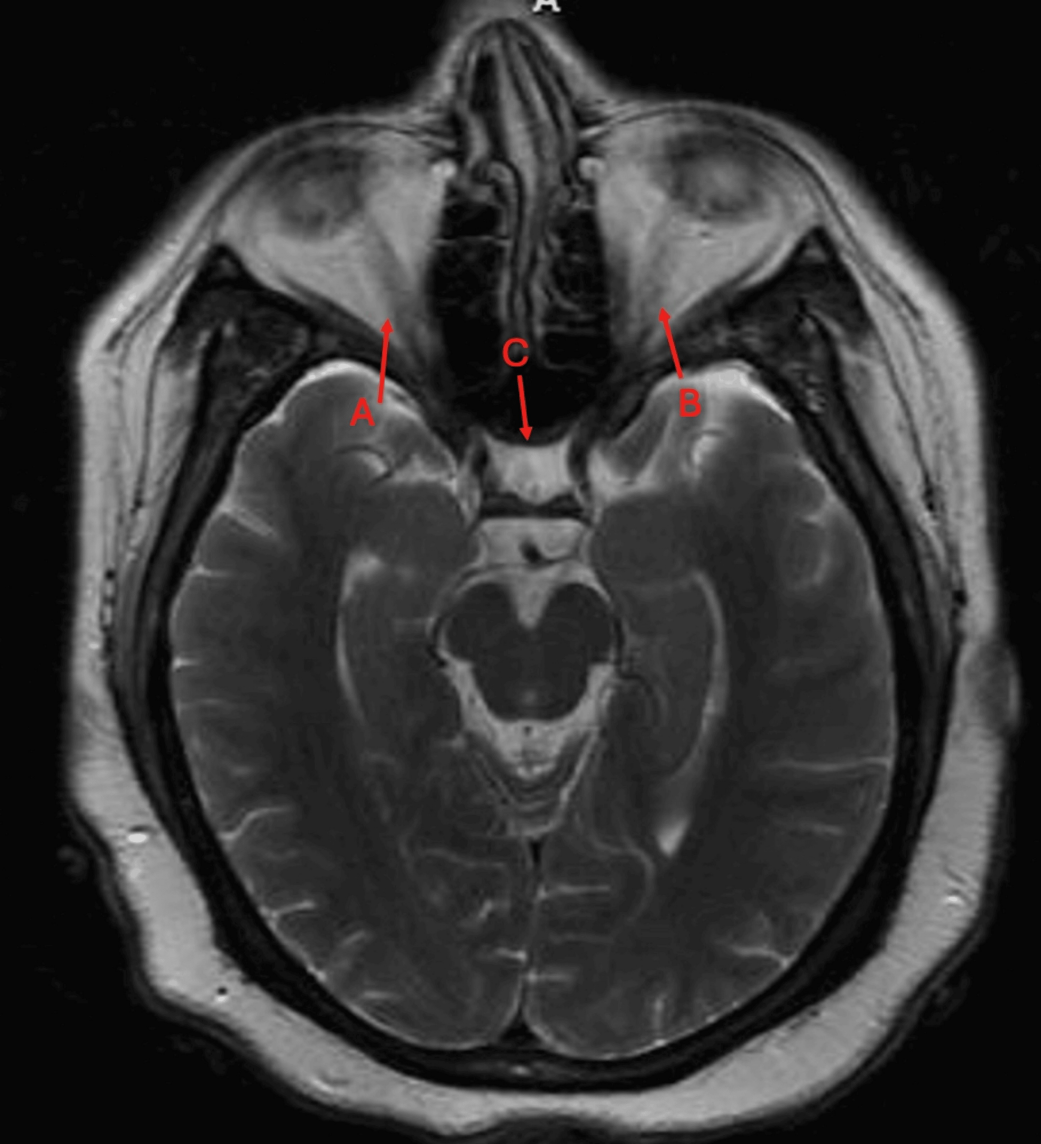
Axial T2-weighted MRI of the brain in a patient with neuromyelitis optica. Arrows A and B indicate the optic nerve. The opaque (white) regions within the nerve are signs of inflammation, i.e., optic neuritis. Arrow C is pointing to the optic chiasm. MRI shows hyperintense signal abnormalities in the optic nerves, most pronounced bilaterally, consistent with inflammation or prior optic neuritis. The findings suggest optic nerve involvement, a common characteristic of neuromyelitis optica. No acute demyelinating lesions are visible within the brainstem or cerebellum at this level. The ventricles appear symmetrical and there are no signs of significant mass effect or midline shift.
